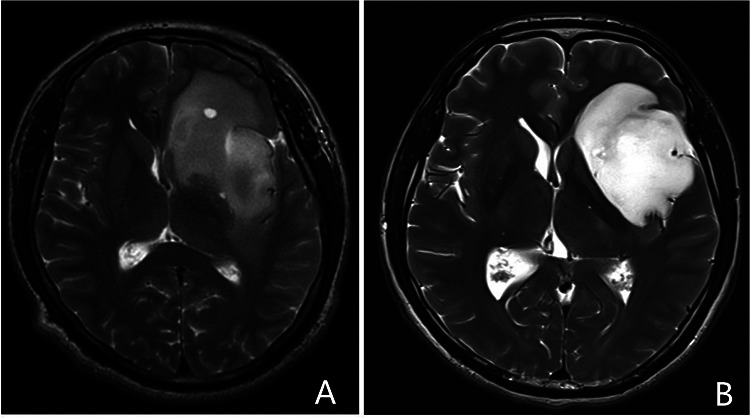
Representative images of the flat inner edge sign of insular gliomas based on the preoperative MRI. (A) Insular glioma with an obscure inner edge sign. (B) Insular glioma with a flat inner sign. MRI, magnetic resonance imaging.
